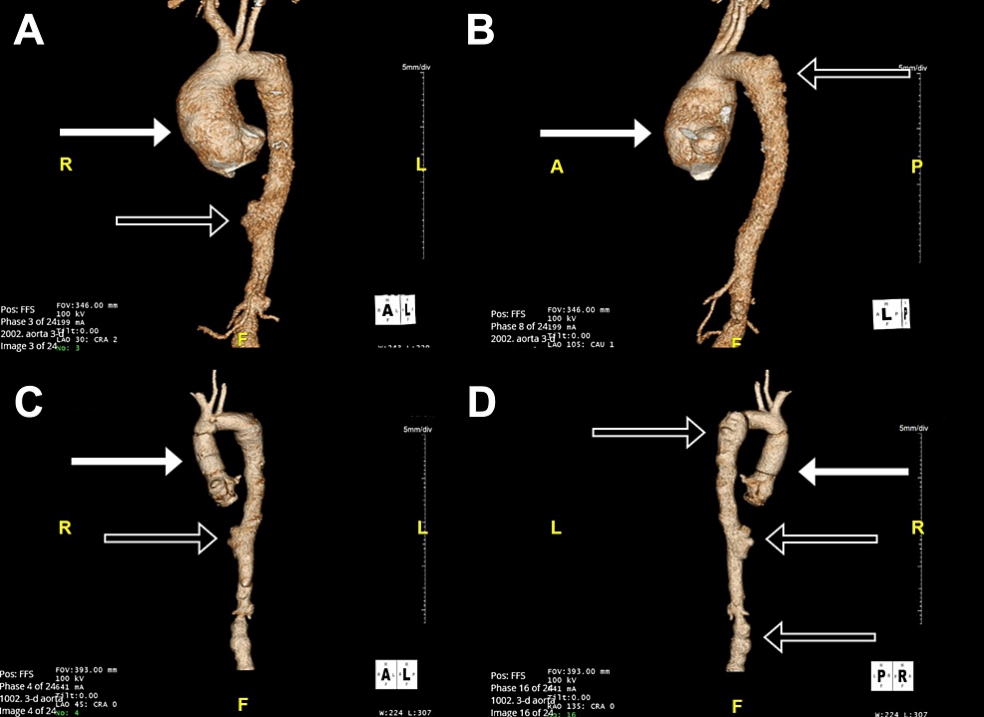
CT angiogram of the chest and abdomen with reconstructed 3D images of the aorta (A) Anterior-posterior 3D reconstructed image of the aorta showing the ascending aortic aneurysm (white arrow) measuring 5.9 cm at maximal diameter. A saccular aneurysm with serrated/irregular contour (black arrow) measuring 3.4 cm at maximal diameter is also present in the distal descending thoracic aorta. (B) Posterior-anterior 3D reconstructed image of the aorta again showing the ascending aortic aneurysm (white arrow) and eccentric dilation of the proximal descending aorta with serrated/irregular contour (black arrow) measuring 3.8 cm at maximal diameter. (C) Anterior-posterior 3D reconstructed image of the aorta showing aortic root graft (white arrow) post-Bio-Bentall procedure. The saccular aneurysm in the distal descending aorta is again visualized (black arrow). (D) Posterior-anterior 3D reconstructed image of the aorta showing aortic root graft (white arrow) post-Bio-Bentall procedure. Multiple saccular aneurysms can be seen in the proximal descending aorta, distal descending aorta, and infrarenal abdominal aorta (black arrows).
